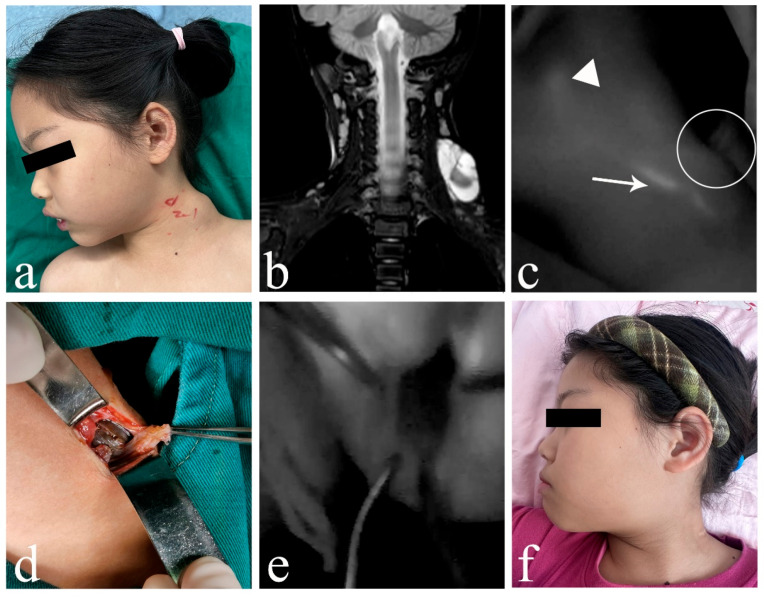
A 7-year-old female patient suffered from mixed cLM with ICH in left neck. (a) Clinical appearance preoperatively. (b) Preoperative MRI indicated mixed cysts with ICH. (c) ICG lymphography showed that one lymph vessel from posterior auricular region bypassed the lesion, and no communication between lymph vessel and the lesion cite was observed. (d) Gross observation of ICH intraoperatively. (e) No ICG was found inside the lesion. (f) Sixteen months postoperatively, complete regression of cLM met the criterion of excellent curative effect. *Arrow head*, lymph vessel; *circle*, region of the lesion; *triangle*, lymph node. cLM, cystic lymphatic malformation; ICH, intracystic hemorrhage; MRI, magnetic resonance imaging; ICG, indocyanine green.
